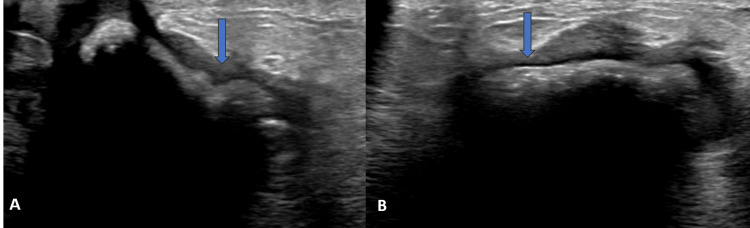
Ultra-sonography images (case two) (A) and (B) show a hypoechoic lesion with a central hyper-echoic wavy structure (arrow), producing dense posterior acoustic shadowing.
